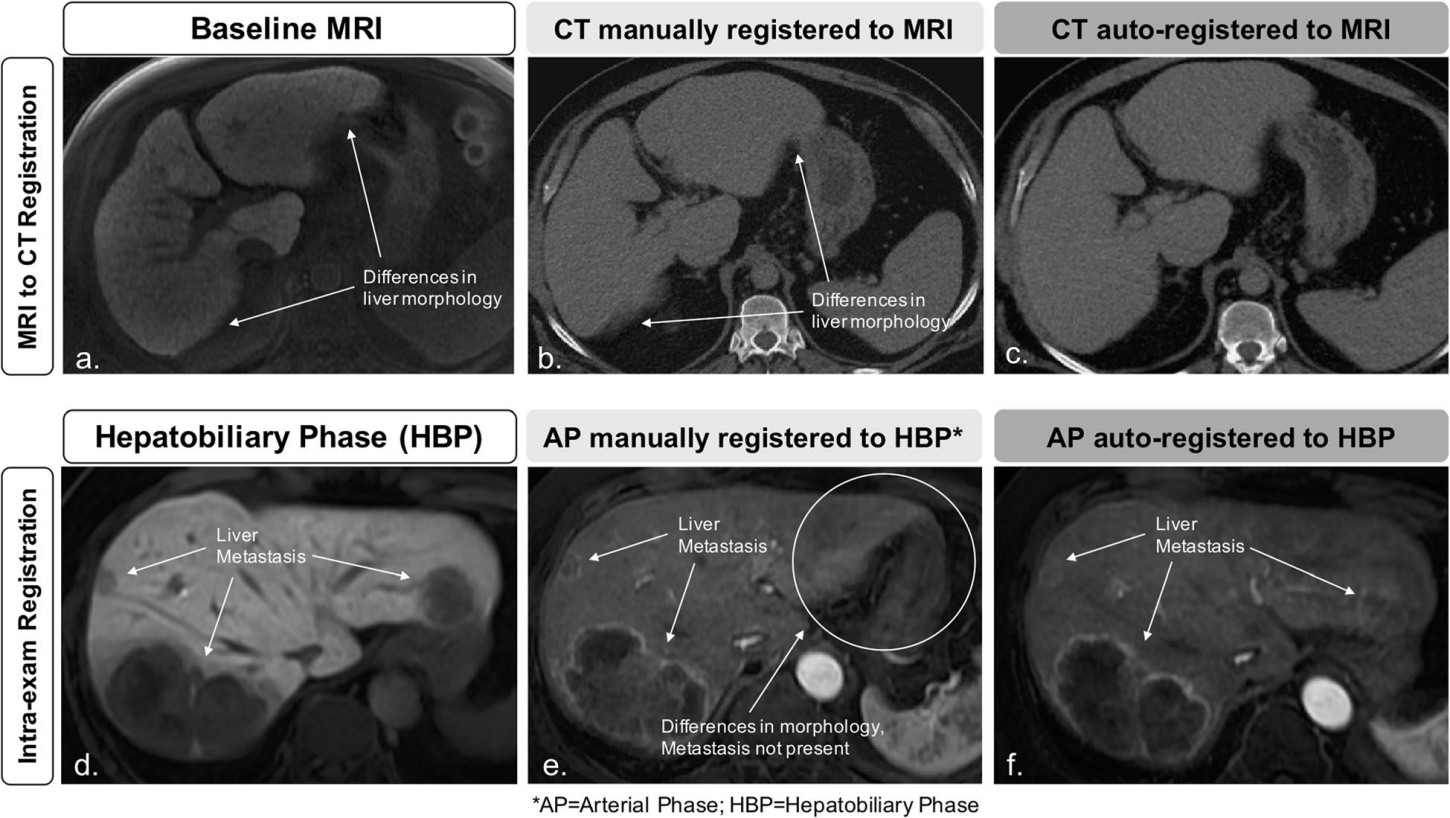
Feasibility of cross-modality and multiphase registration. The registration algorithm (using binary masks) was applied to a cross-modality series pair (computed tomography to magnetic resonance imaging; a–c) and one intra-exam multiphase series pair (HBP to arterial phase; d–f) as proof-of-concept examples of cross-modality and multiphase registration, respectively
